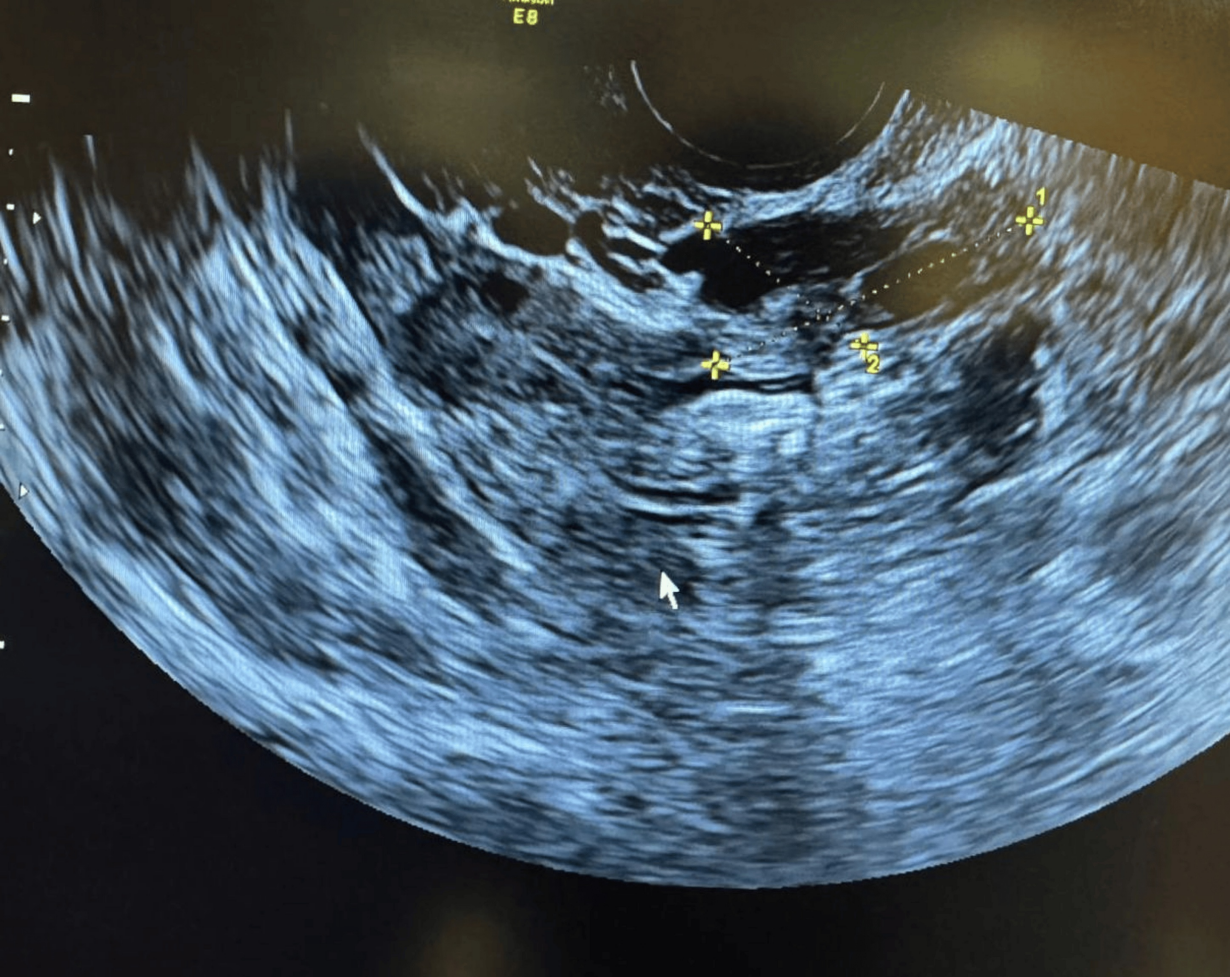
Ultrasonography of the left ovary after laparoscopic surgery
